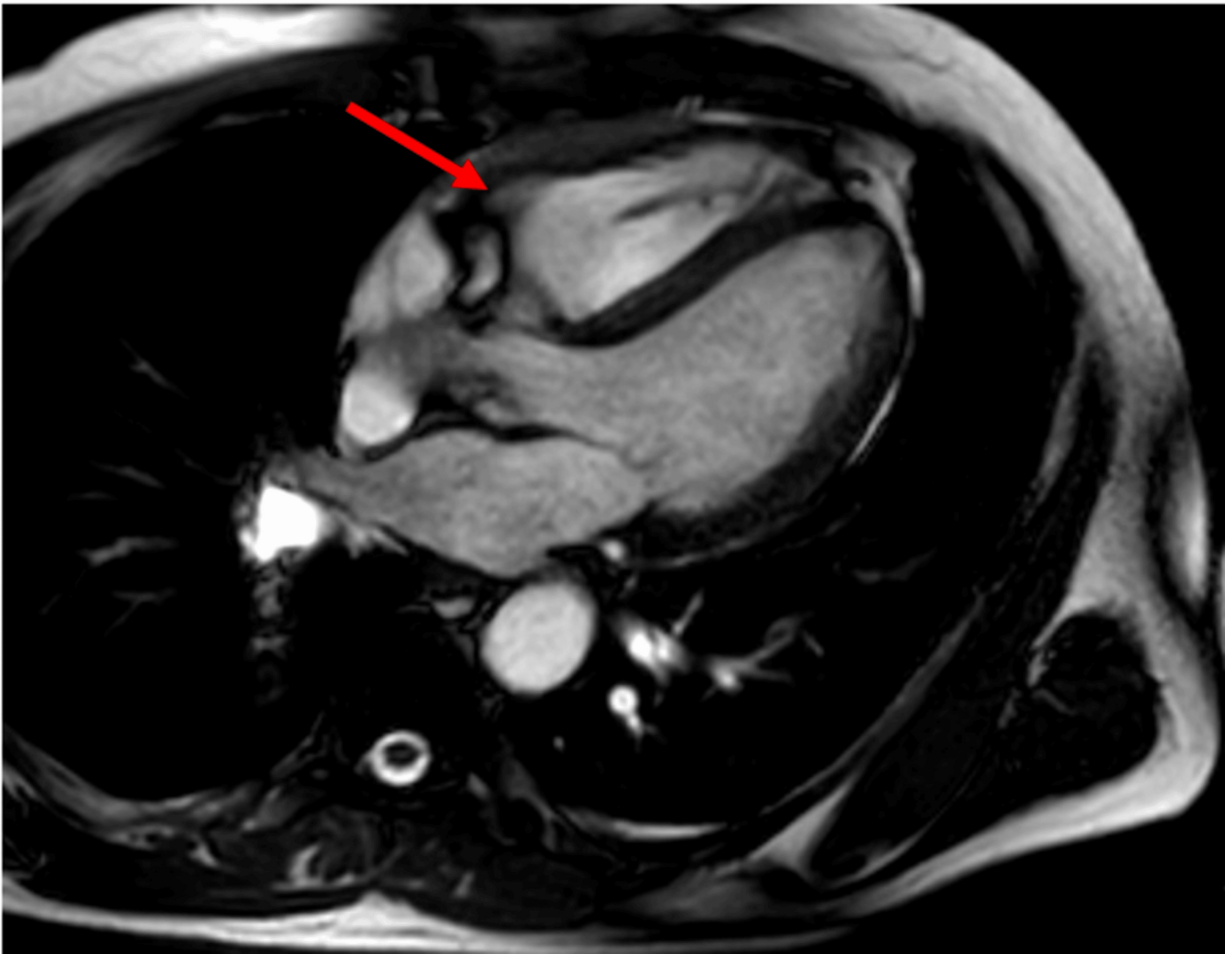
Cardiac MRI showing an ill-defined mass in the right ventricle (red arrow)
